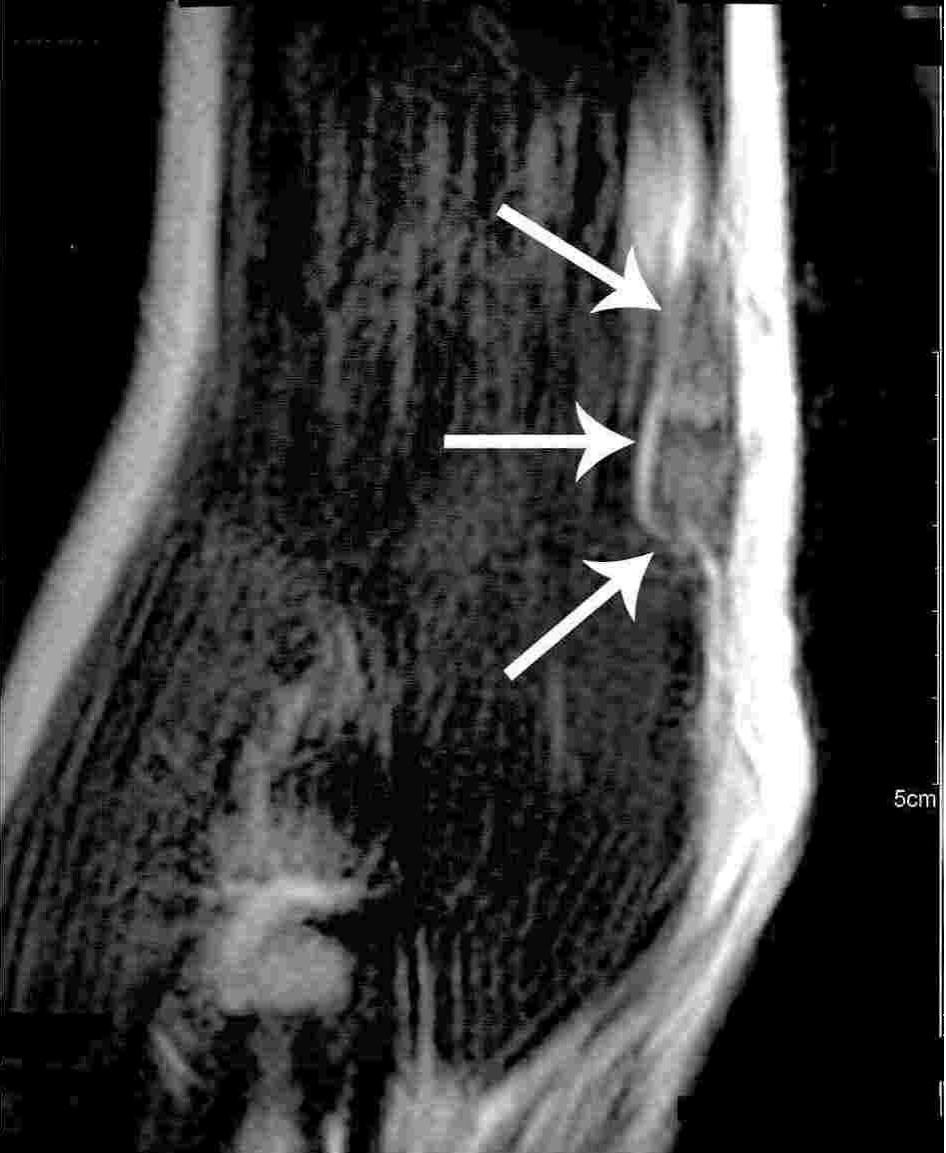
Coronal T2-weighted MRI reveals a slightly hyperintense fusiforme tumorous lesion of the median nerve approximately 5 cm above the right elbow (arrows).
